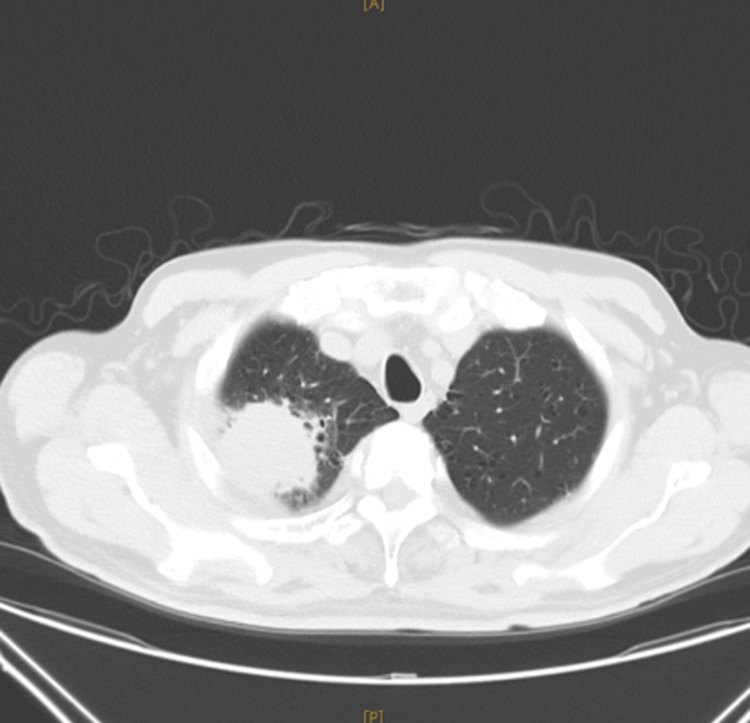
Computed tomography (CT) before resection of lung cancer showing a large mass in the right upper lung
